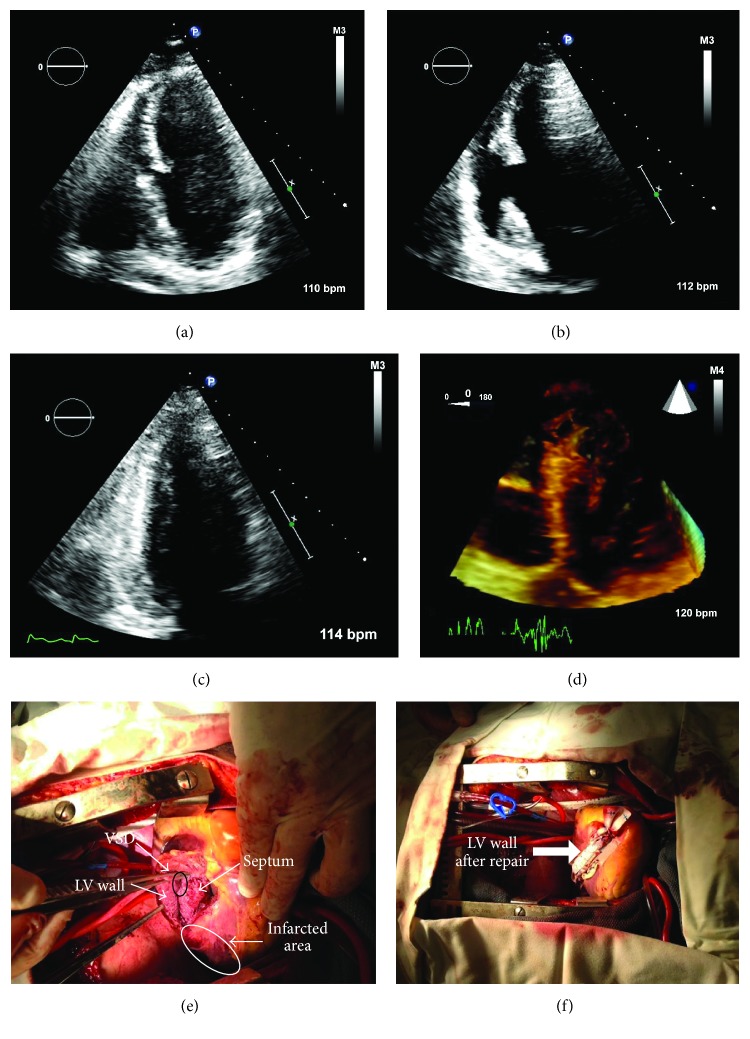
Four-chamber echocardiographic views of an apparent ventricular septal defect of the inferior septum (a) and an apparent pseudoaneurysm of the inferior wall (b), which persisted despite rotating the transducer 90 degrees or more from the four-chamber apical window. On repeat imaging, a very narrow apical window was identified in which the two-chamber view was seen without the pseudoaneurysm (c). Also shown is a three-dimensional echocardiographic depiction of the serpiginous nature of the ventricular septal dissection (d). Surgical view of the infarcted area and partial ventricular septal defect (e). Surgical view of the septum covered by a Dacron patch (f).
